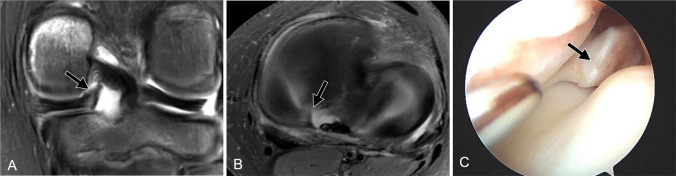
Medial meniscus posterior root avulsion in a 10-year-old boy. Coronal proton-density-weighted fat-suppressed MRI (A), axial proton-density-weighted fat- suppressed MRI (B), and arthroscopic view (C) demonstrate complete avulsion of the posterior root of the medial meniscus (arrows) at the enthesis
